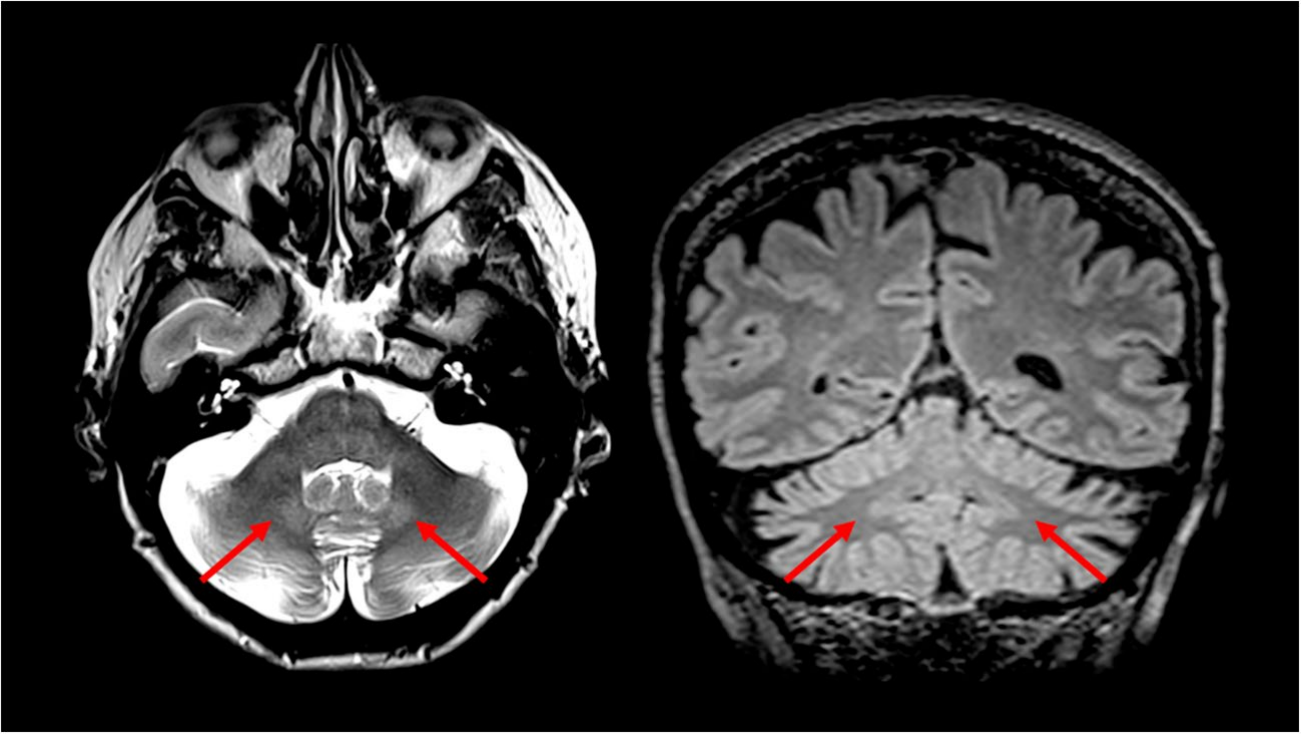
An example of Dentate Nuclei T2w hyperintensity. Axial T2-weighted (*left*) and coronal multiplanar reconstruction of a volumetric Fluid Attenuated Inversion Recovery sequence (*right*) of a 70-year-old female Spastic Paraplegia Type 7 patient showing a bilateral T2-weighted hyperintensities of the Dentate Nuclei (*red arrows*)
